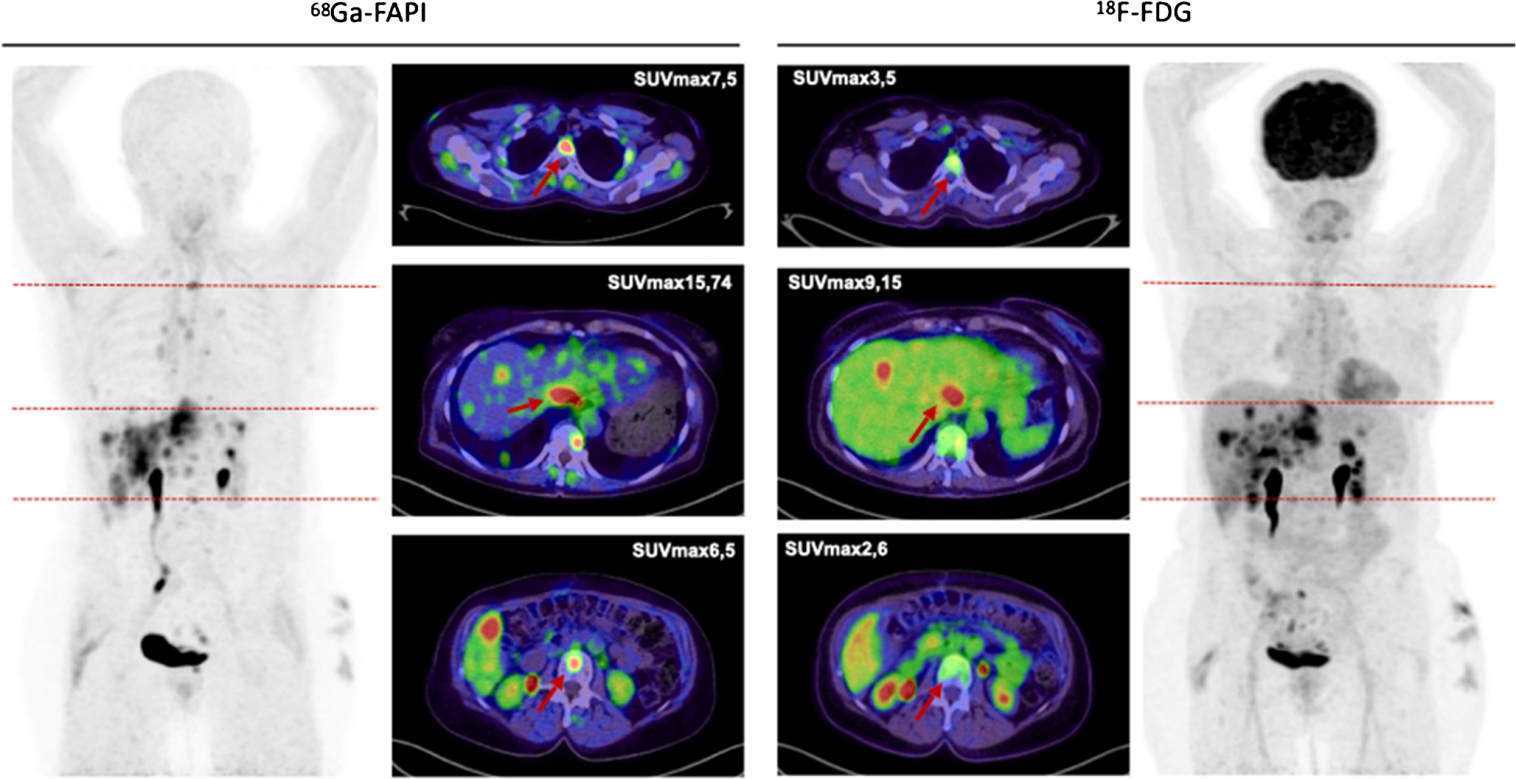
A 63-year-old woman with metastasized ovarian carcinoma underwent ^68^Ga-FAPI-PET/CT followed by ^18^F-FDG-PET/CT 1 month later. Tracer uptake in normal liver parenchyma was markedly different in both tracers: ^68^Ga-FAPI SUVmax 1.38 vs. ^18^F-FDG SUVmax 4.34. The quantified uptake in two bone metastases and one liver metastasis presented a rather high FAPI-uptake compared to FDG, respectively
